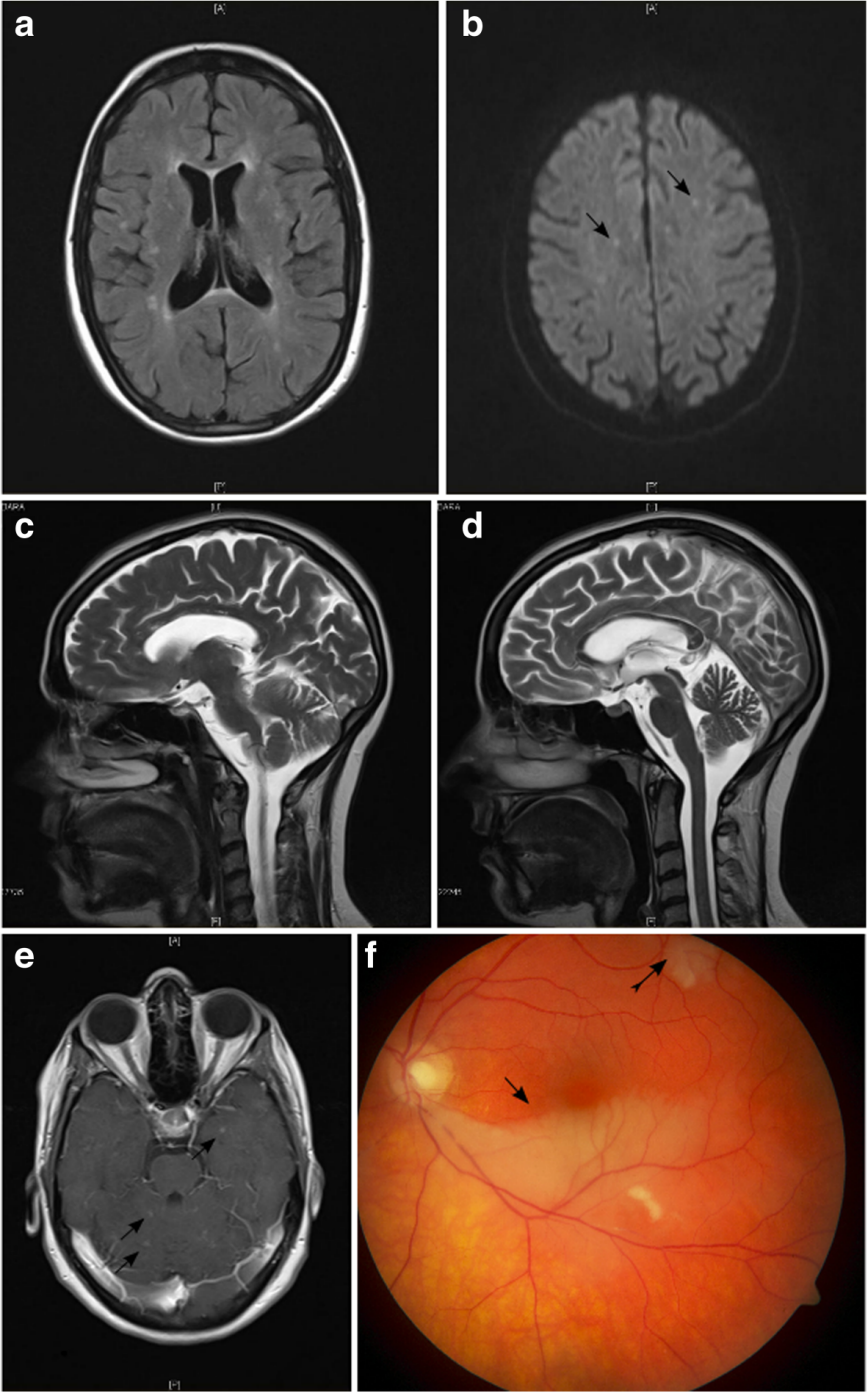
MRI, DSA and funduscopic studies.a) Axial fluid-attenuated inversion recovery image showing multiple small white matter lesions. b) Diffusion-weighted image with multiple small lesions exhibiting diffusion restriction (arrows) and correspondingly decreased ADC values (not shown). c) Sagittal T2 image showing centrally located corpus callosum lesions (arrow) and one lesion with spoke-like morphology (tailed arrow). d) Near-median sagittal T2 image demonstrating atrophy of the corpus callosum. e) Contrast-enhanced T1 image with multiple small contrast-enhancing lesions (arrows). f) Large ischemic area in the lower half of the left retina (arrow) and smaller infarct in the top left quadrant (tailed arrow).
